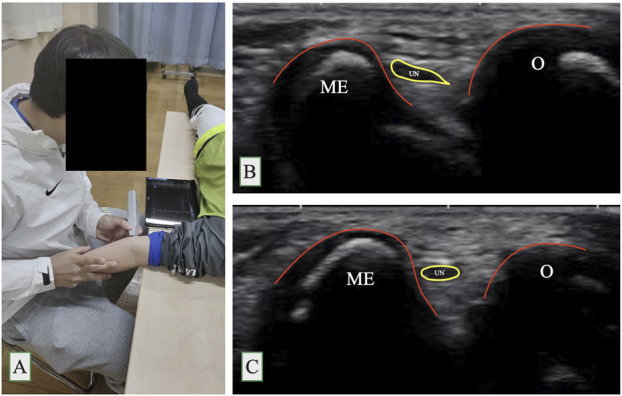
Standardized positioning and transducer protocol for dynamic ultrasonographic evaluation of the UN. Fig. 1-A A board-certified orthopedic surgeon performing dynamic ultrasonography on a child in the standardized supine position with the shoulder abducted and elbow flexed according to the study protocol. Fig. 1-B The transducer is placed transversely between the ME and olecranon to obtain a short-axis view of the UN within the cubital tunnel. Fig. 1-C The probe is slid to visualize the apex of the ME and then tilted until the ultrasound beam is perpendicular to the UN, obtaining an optimized short-axis image with circular or elliptical morphology. ME = medial epicondyle, O = olecranon, and UN = ulnar nerve.
